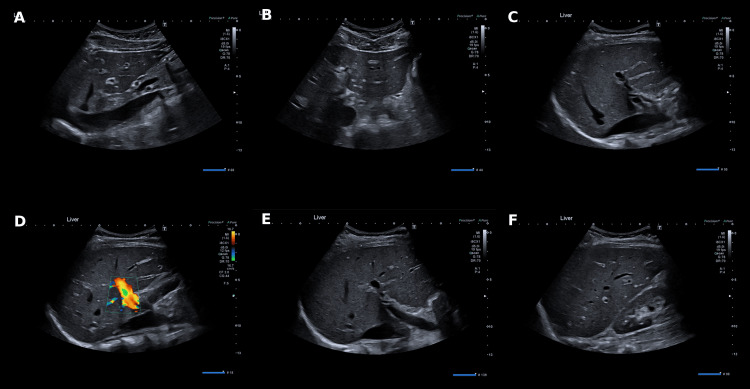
Abdominal ultrasound demonstrating normal hepatic architecture and biliary anatomy Transverse and longitudinal ultrasound images (A–F) of the upper abdomen.
